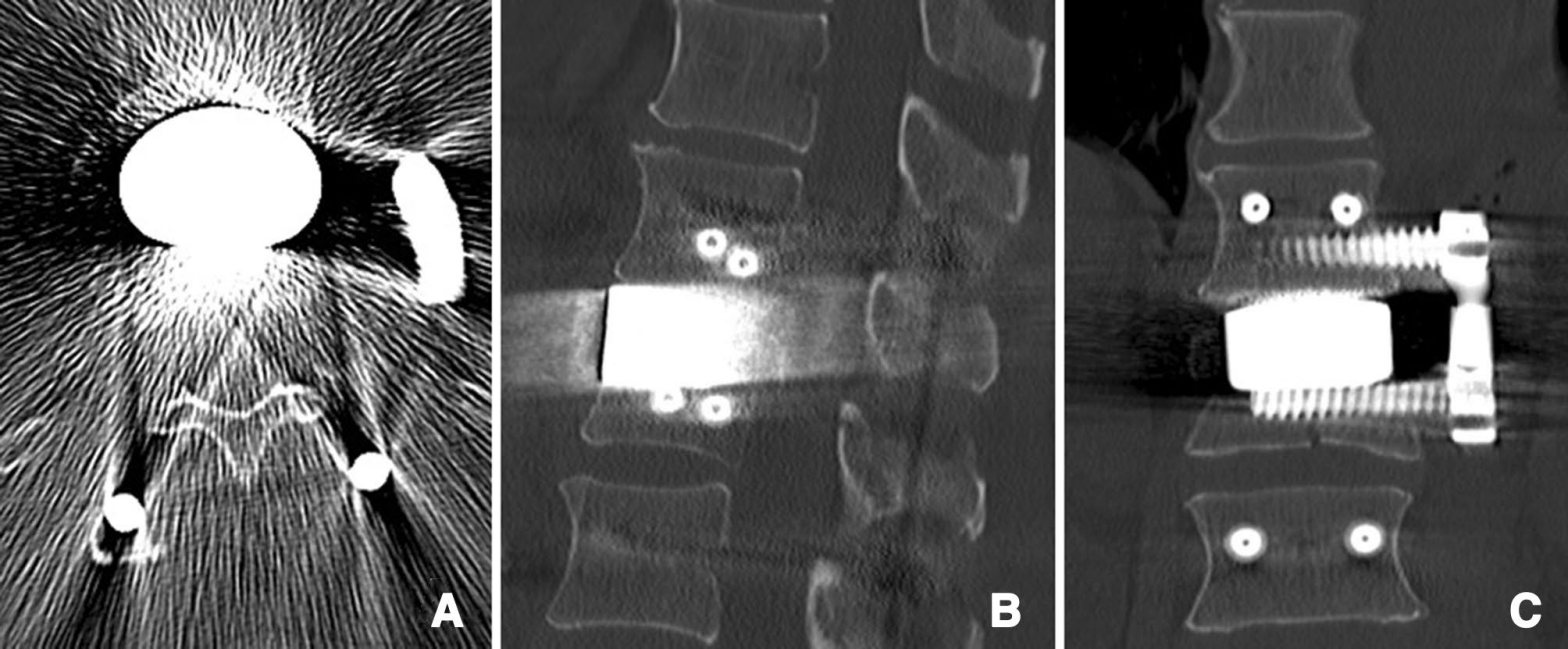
Postoperative CT of a 65-year-old man with a superior burst-split fracture of L1 (Magerl et al.^19^ 3.2.1, AOSpine A4^1^) treated by short segment posterior instrumentation and anterior one-level spondylodesis using a porous tantalum fusion implant (PTFI). Representative axial (A), sagittal (B) and coronal (C) slices.
